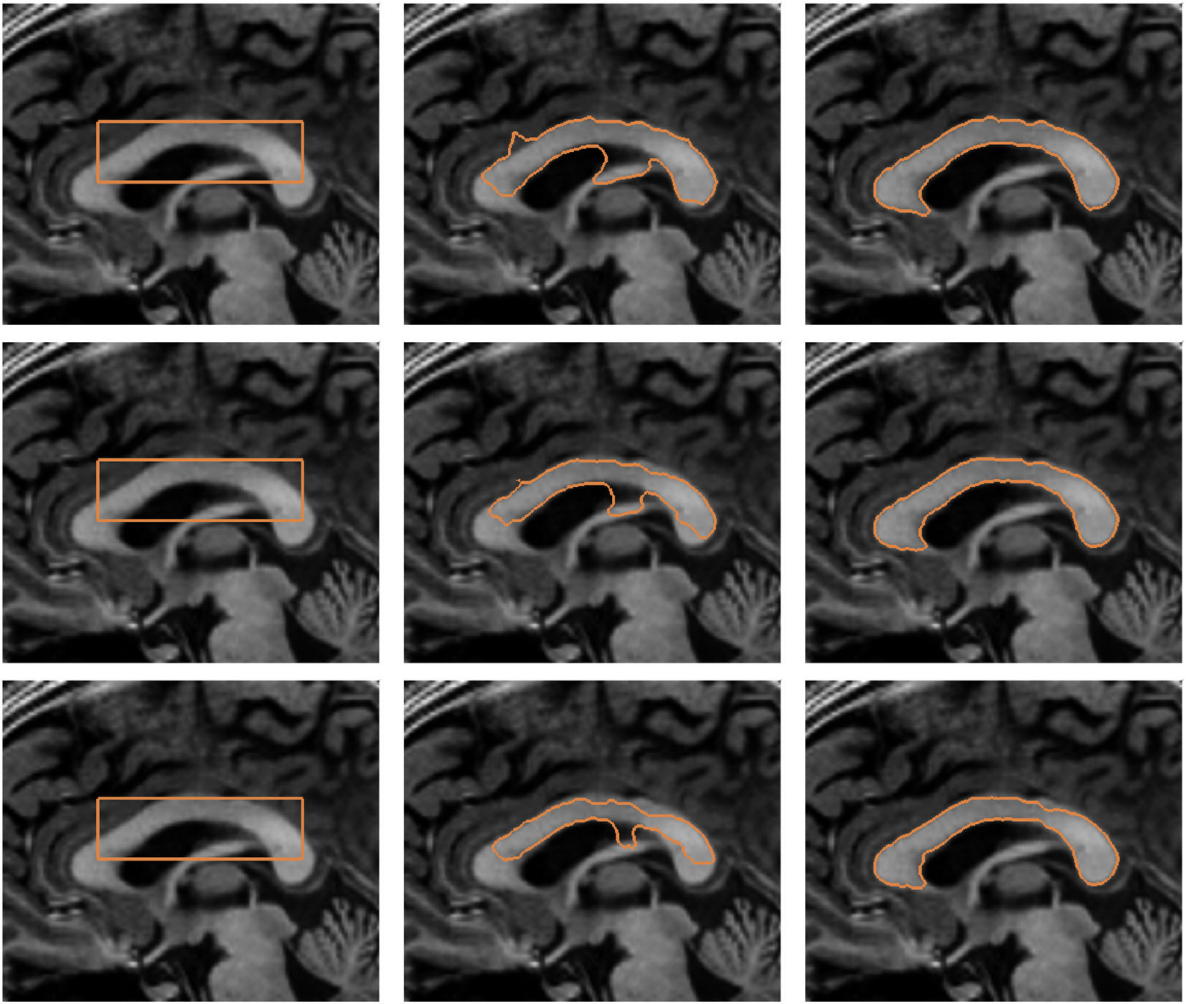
Segmentation of corpus callosum from magnetic resonance image using the proposed active contour with shape prior using different parameters: (top row) *ν* = 2.0 × 10^4^, (middle row) *ν* = 3.5 × 10^4^, and (bottom row) *ν* = 5.0 × 10^4^.
